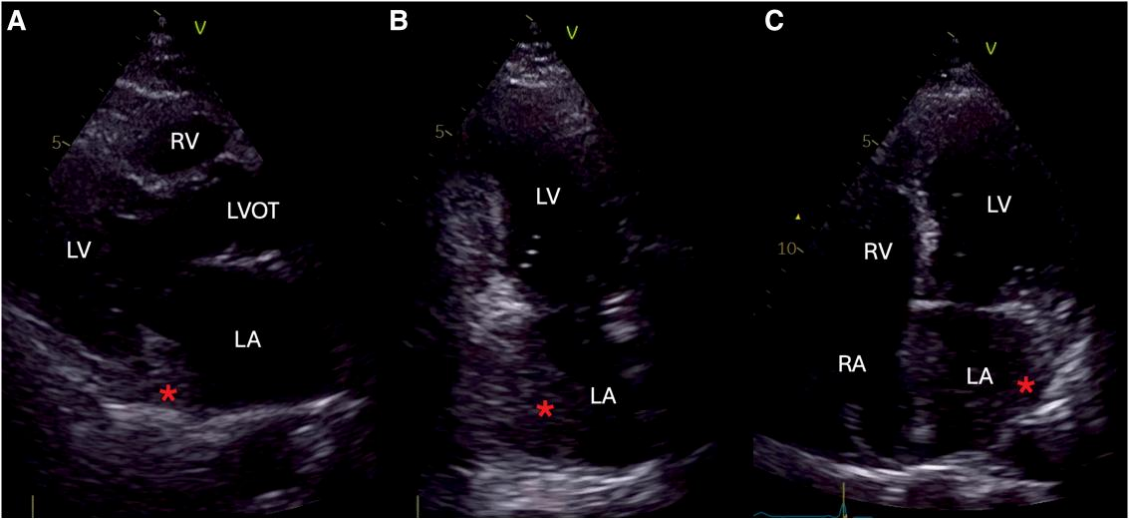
Transthoracic echocardiography shows a hypoechogenic mass (asterisk) occupying the posteroinferior wall of the left atrium, without pedunculation or mobile components. (*A*) Parasternal long-axis view. (*B*) Apical two-chamber view. (*C*) Apical four-chamber view.
